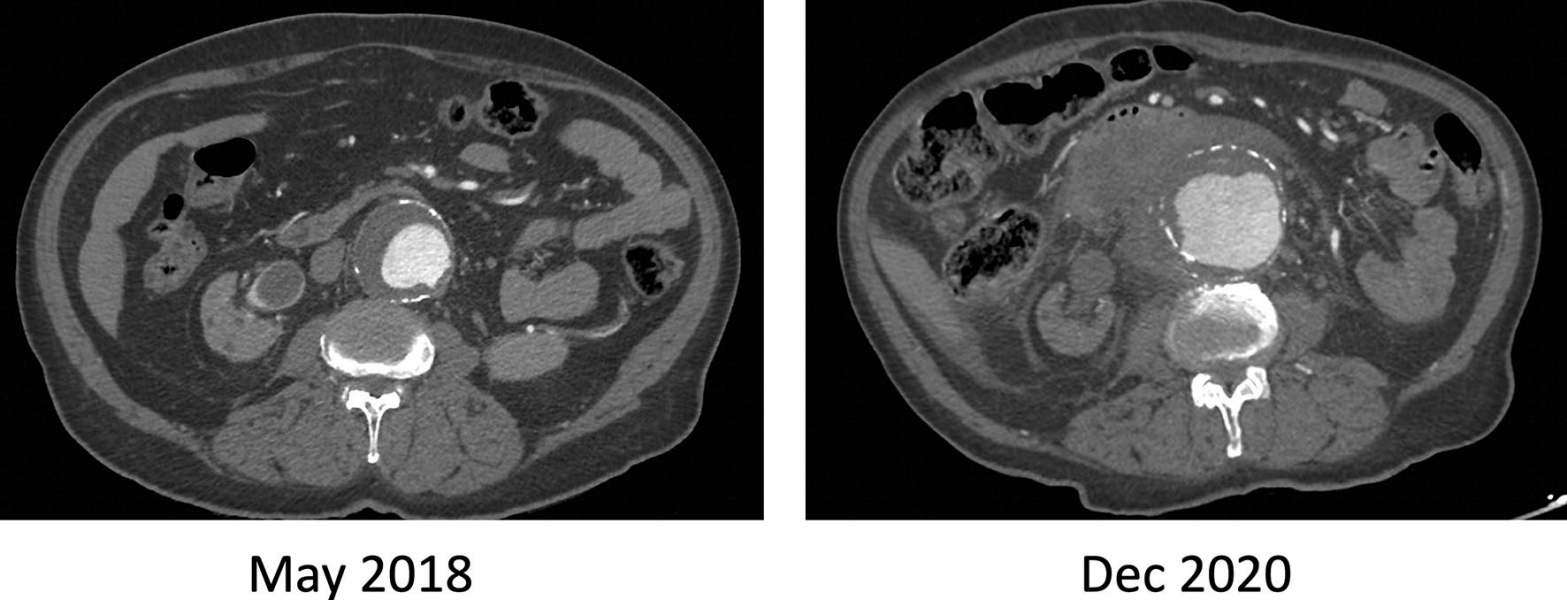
CT scan with contrast comparing 2017 and 2020 showed marked expansion of abdominal aortic aneurysm with evidence of retroperitoneal bleeding
